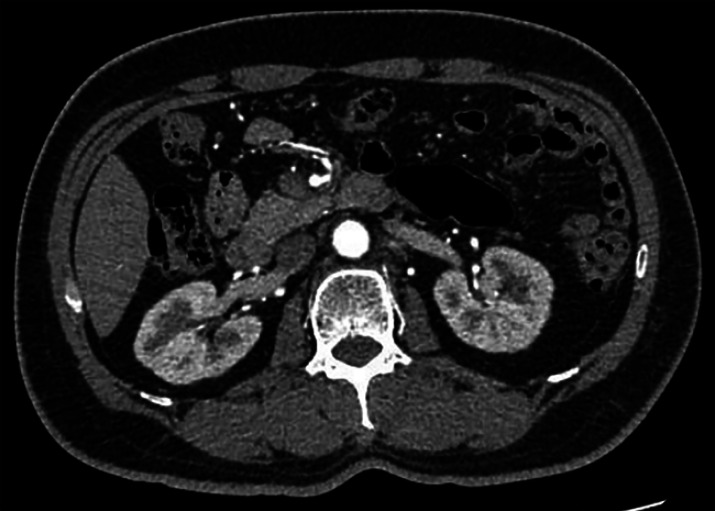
Enhanced computed tomography showing thickening of the duodenal horizontal wall.
